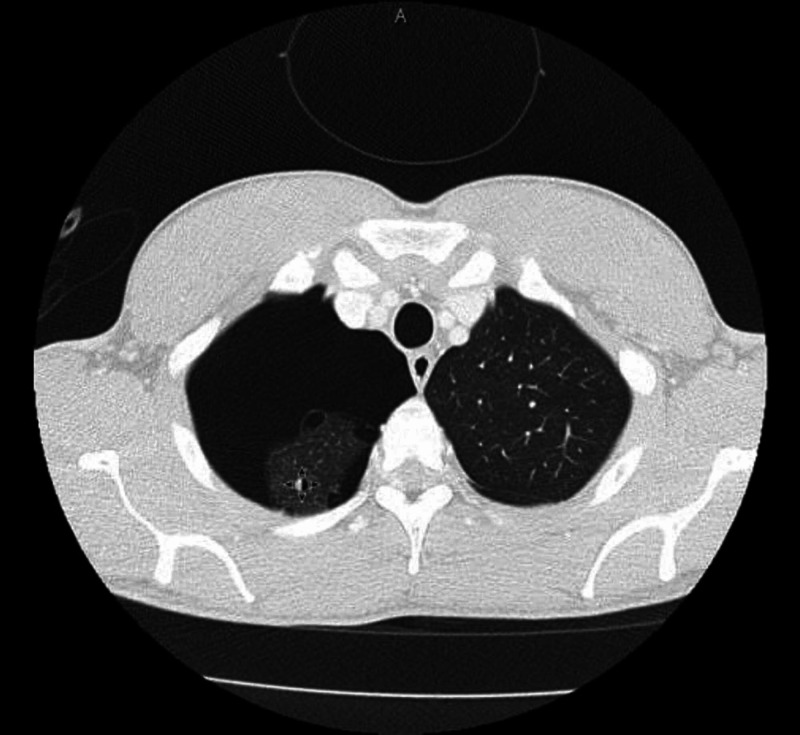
CT-thorax shows large right-sided pneumothorax with several small pleural blebs at the right apex and margin of the right upper lobe.
